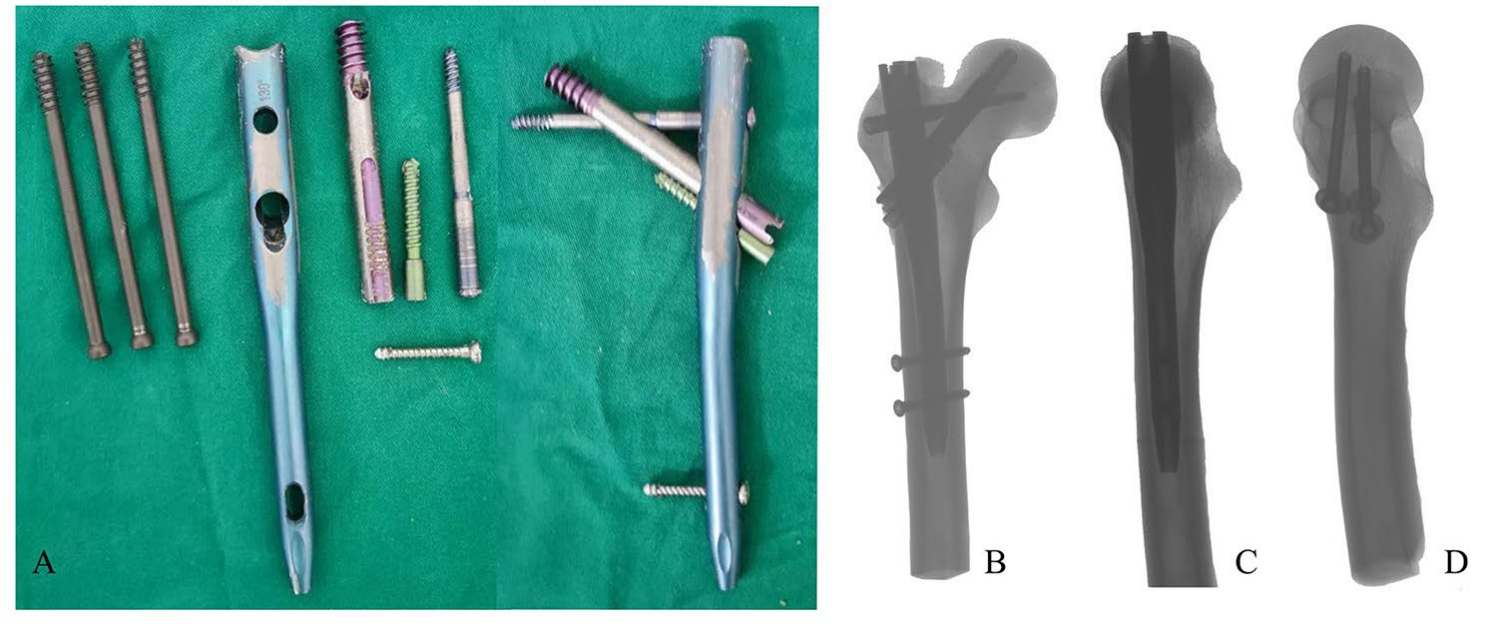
Structure of PFBN and ITCS(A) and radiographs of femoral neck fractures fixed by PFBN and ITCS(B – C)
